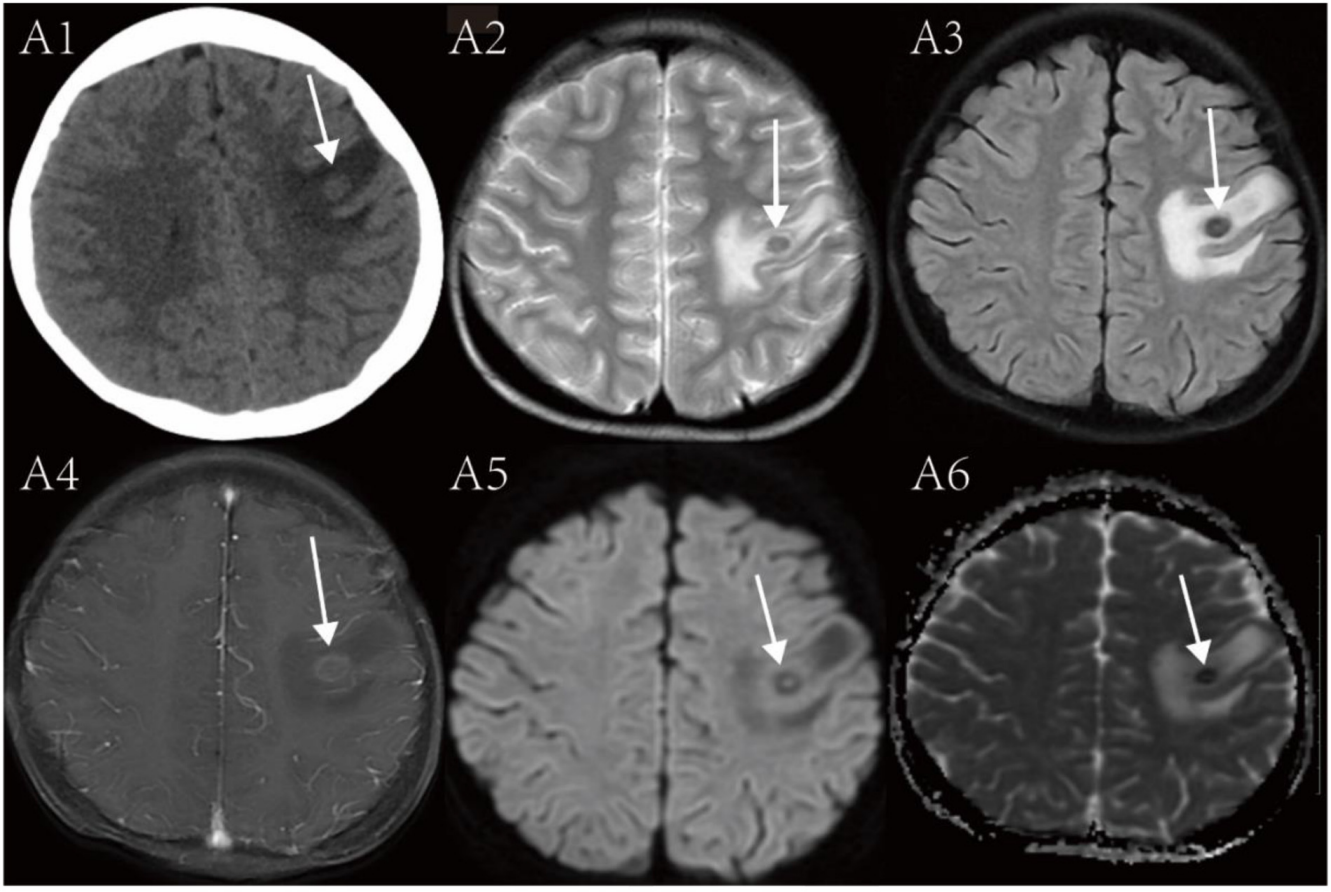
Brain imaging findings for patient 1 upon presentation. (A1) Non-contrast CT image showing a slightly low-density nodular lesion in the left frontal lobe on (white arrow) with surrounding low-density edema. (A2) T2-weighted image showing the left frontal lobe lesion with a three-layered target sign of low-high-low signal from the periphery to the center as seen on (white arrow). (A3) T2-fluid attenuated inversion recovery (FLAIR) image showing a nodular central punctate high signal surrounded by low signal in the left frontal lobe as seen on (white arrow). (A4) The contrast-enhanced T1 image showing the ring enhancement of the lesion (white arrow). (A5) Diffusion weighted imaging (DWI) showing the left frontal lobe lesion with a central dot of isointensity surrounded by a ring of hypointensity and then a ring of isointensity. (A6) Apparent diffusion coefficient (ADC) image showing the left frontal lobe lesion with a central point of high signal surrounded by a ring of low signal with associated edema.
